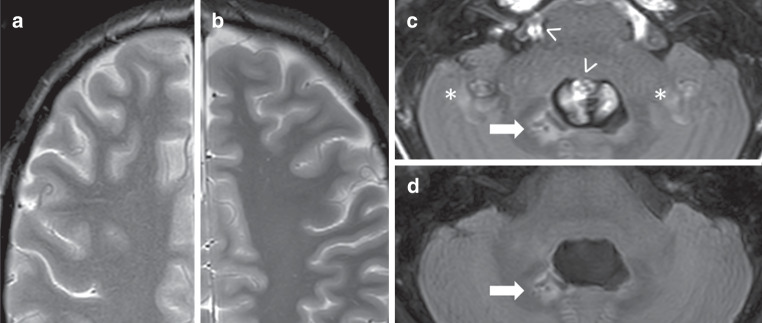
Comparison of T2-TSE and FLAIR images obtained with the standard and C‑SENSE pediatric brain tumor protocols. Example from a 6.4-year-old male patient with infratentorial astrocytoma. Images of the frontal lobes obtained with the T2-TSE sequence in the standard (a) and C‑SENSE (b) protocols. Note the noisier appearance of the standard examination and similarity of the contrast of gray and white matter and sharpness of smaller structures (e.g., cortical vessels) between sequences. Images of the posterior fossa obtained with the FLAIR sequence in the standard (c) and C‑SENSE (d) protocols. Due to T1 adjustment, CSF flow artifacts (*arrowhead*) are suppressed sufficiently and their ghosts (*asterisk*) are eliminated in the C‑SENSE image. Pathology is present after astrocytoma resection (*arrow*)
